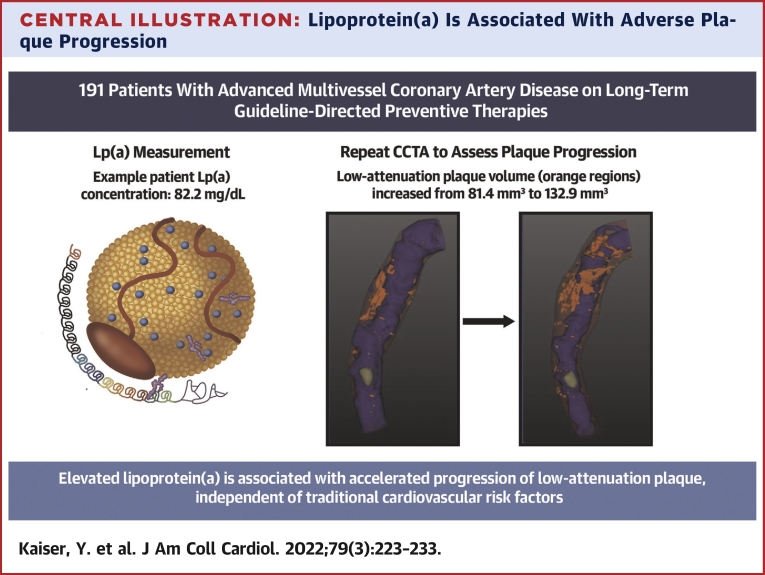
Lipoprotein(a) Is Associated With Adverse Plaque Progression Patients with advanced multivessel coronary artery disease with elevated Lp(a) concentrations (≥70 mg/dL) showed accelerated progression of low-attenuation plaque. An example patient with elevated Lp(a) (82.2 mg/dL) showed marked progression of low-attenuation plaque volume (visualized in orange): increased from 81.4 to 132.9 mm^3^ after 1-year follow-up. CCTA = coronary computed tomography angiography; Lp(a) = lipoprotein(a).
